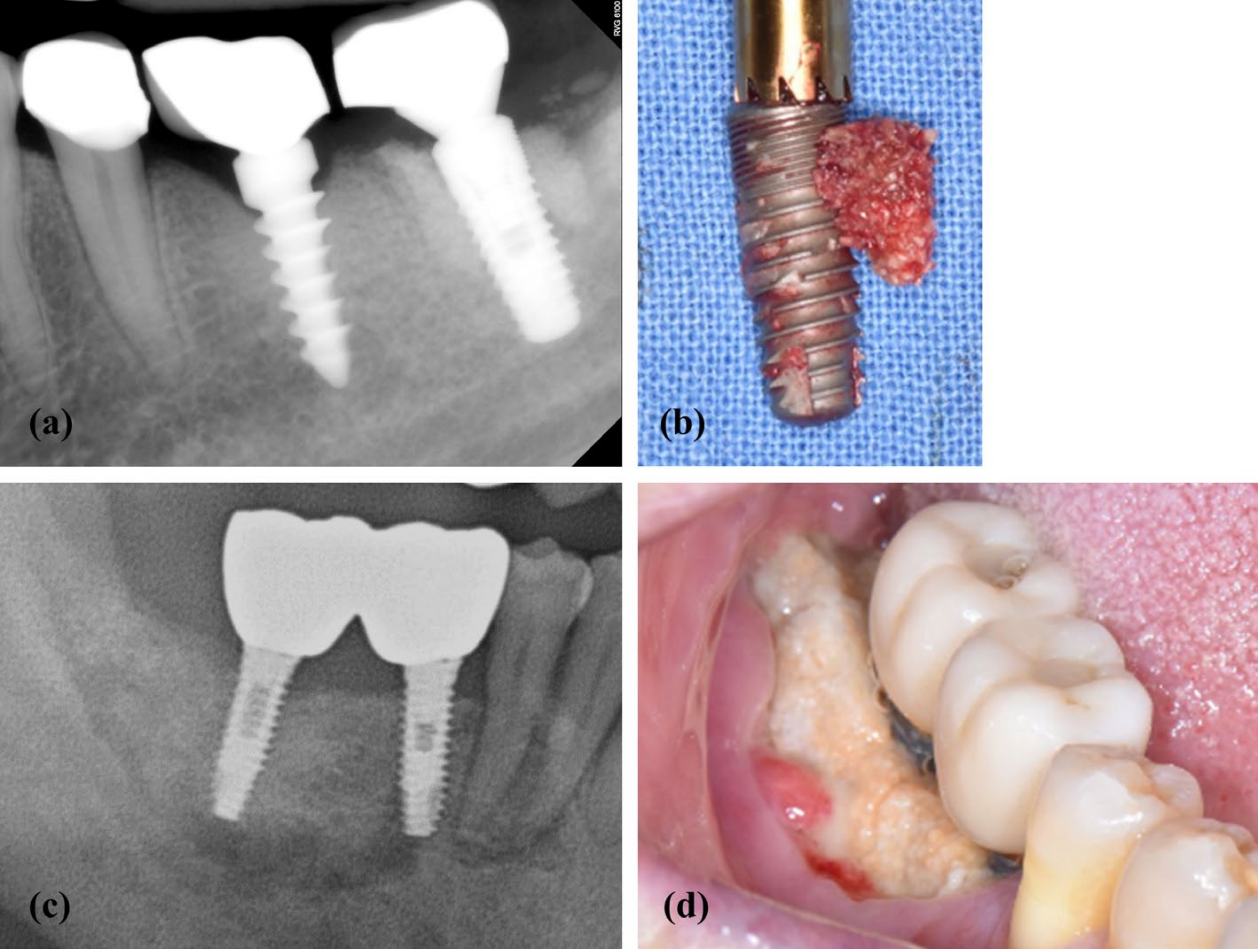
Affected lesion difference according to the type of prosthesis. (a) Preoperative radiographic view of a single implant supported crown. (b) Postoperative clinical photograph of a single implant supported crown peri‐implant medication‐related osteonecrosis of the jaw (PI‐MRONJ). (c, d) Preoperative radiographic view and clinical photograph of a splinted implant‐supported crowns.
